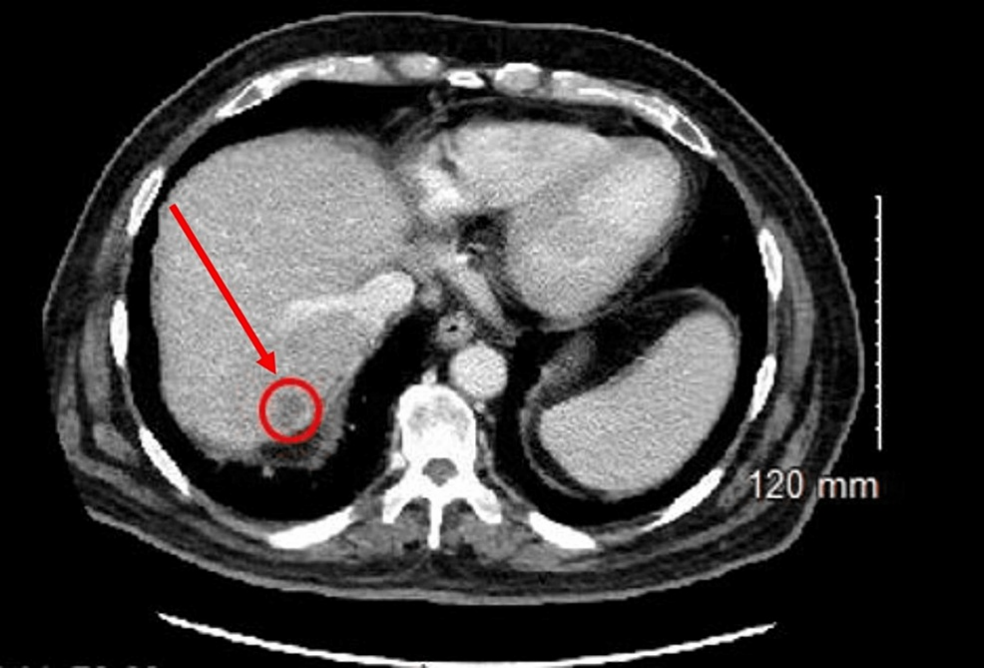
Axial CT of the abdomen and pelvis Axial CT of the abdomen and pelvis visualizes the hypodense lesion previously treated by 90Y segmentectomy (encircled in red) in hepatic segment VII with some peripheral enhancement.
